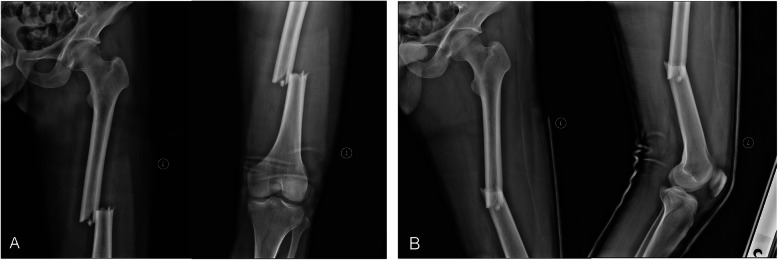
View of a 36-year-old male with a 32A3 fracture. a Preoperative anterior-posterior X-rays. b Preoperative lateral X-rays
